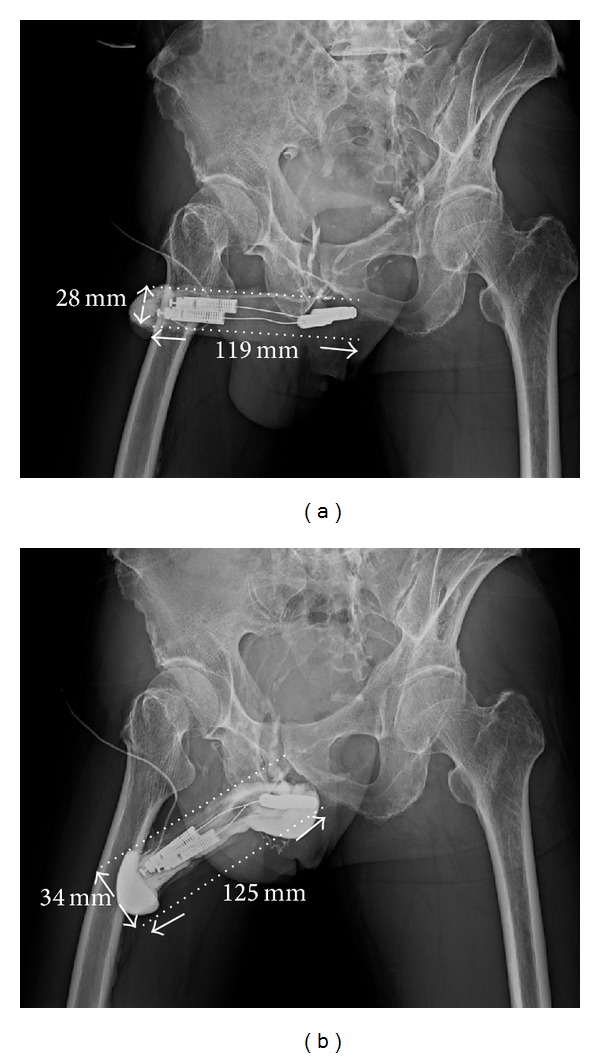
Pelvic X-ray film of 30° oblique view of a 65-year-old male. He underwent the first surgery somewhere in 2005. A cold glans syndrome prompted to receive the venous ligation surgery. (a) The glanular radius was enhanced from 28 mm to 34 mm after the penile venous surgery. The corporeal length was 119 mm from X-ray, and it was 180.0 mm from implant surgery; however 90.0 mm × tan⁡ 60° (1.73205080757) = 206.1 mm. (b) The DDV was ligated at the level of retrocoronal and hilum region. Enhancement was demonstrated in both the glans penis and entire penile shaft after a contract medium was injected to the glans penis via a #23 scalp needle.
